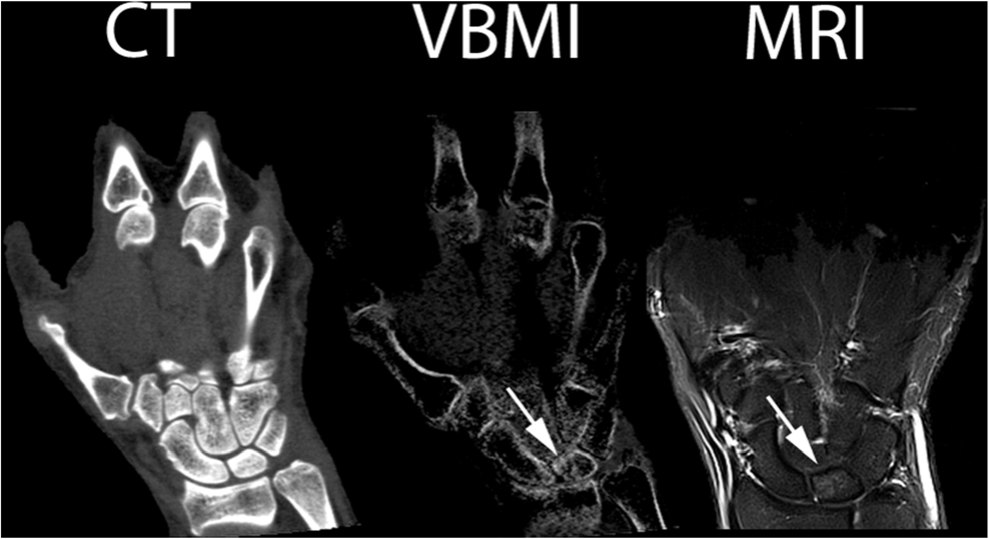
Right wrist of patient No. 7 (CPPD). CT: normal CT image at 135 kV, VBMI: corresponding VBMI image, MRI: corresponding short tau inversion recovery sequence – MRI shows a faint BME in the lunate (*arrow*) that was missed by reader 1. VBMI also displays a slight hyperdensity, which was scored negative by both readers because even normal carpal bones yield increased density in VBMI compared to long bones according to our clinical experience
